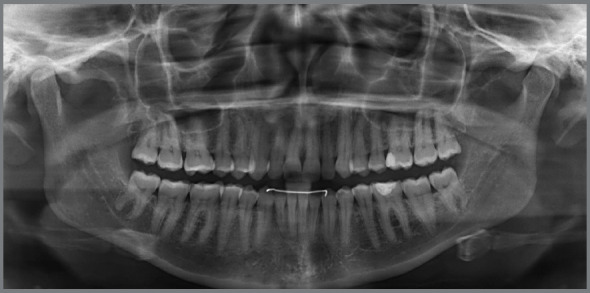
Panoramic radiograph 10 years after the end of treatment.
